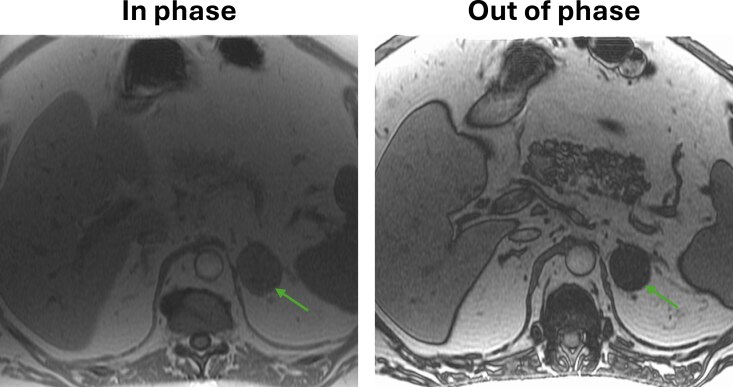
MRI of the adrenal glands performed for case 1 at 12 years after initial lesion detection, demonstrating signal drop out on out-of-phase chemical shift imaging in-keeping with a left-sided, lipid-rich adenoma (green arrows).
